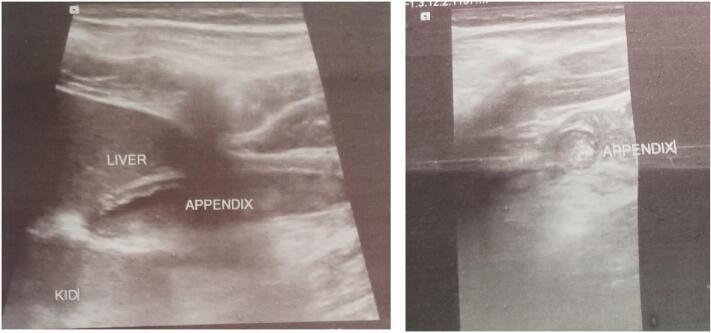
Abdominopelvic ultrasound showing an enlarged non-compressible appendix measuring 9 mm with edematous and thick wall, and fat stranding.
